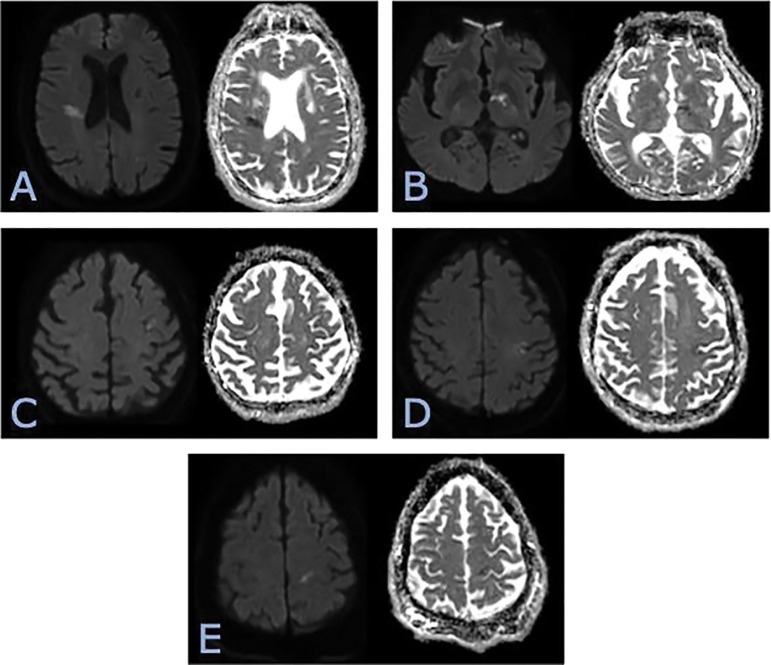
Diffusion weighted MRI images in the axial plane of multiple patients with small acute infarcts with NIHSS of zero including - (A) right corona radiata, (B) left basal ganglia, (C) posterior left frontal lobe cortex, (D) posterior left frontal lobe cortex, (E) posterior left frontal lobe cortex.
